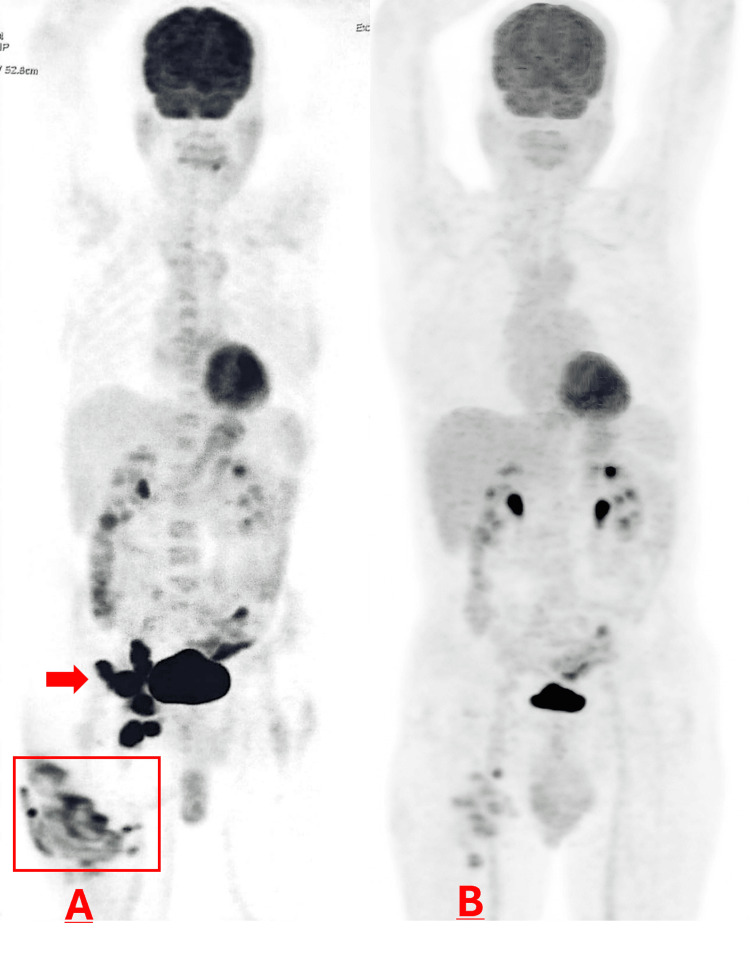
Positron emission tomography/computed tomography findings before and after treatment. (A) Coronal image demonstrating a hypermetabolic right superficial inguinal nodal conglomerate (SUVmax 19.10) and a right internal iliac lymph node (SUVmax 15.08) (arrow). Focal cutaneous and subcutaneous hypermetabolism in the posterior proximal right thigh (SUVmax 9.07; square). (B) Coronal image at follow-up after second-line treatment demonstrating complete metabolic response.
